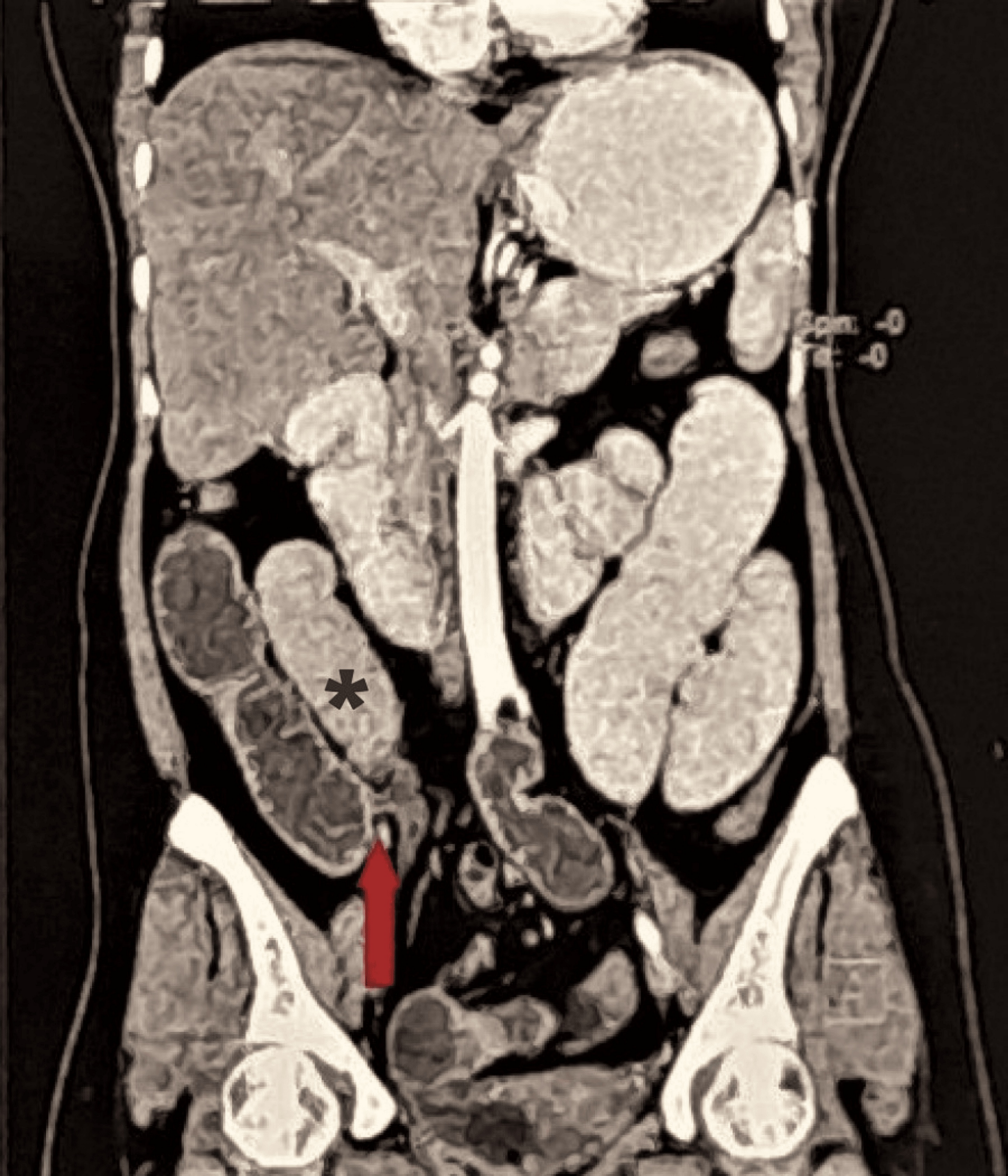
CT of the abdomen and pelvis showing bowel constriction (point of transition with kinking of the bowel) (red arrow) in the right paramedian region. The dilated bowel loop proximal to the constriction (black asterisk) is particularly noteworthy.
